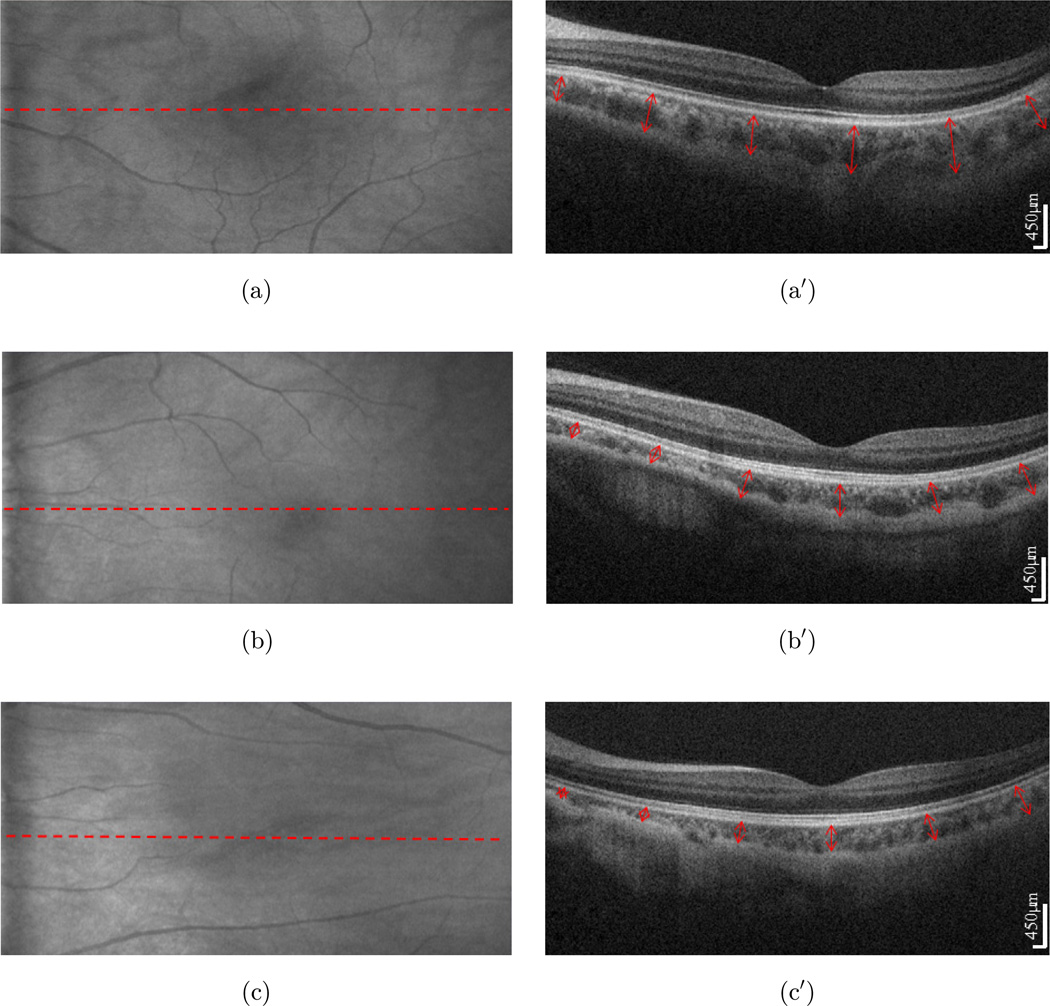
The CT of myopic eyes. (a), (b) and (c) is the projection of the low (−1D), moderate (−5D) and high myopic (−8D) eye, respectively. The choroid is shown on the cross section SDOCT scan (a′) (b′) and (c′). The CT is measured vertically from the outer border of the RPE to the outer border of the choroid (shown with red arrow).
